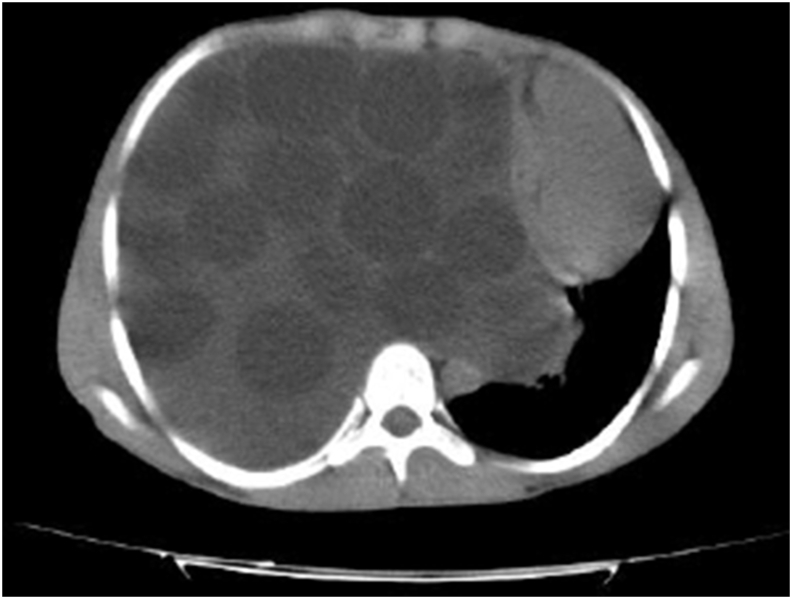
Patient's CT scan: multiple round-shaped cystic formations filling up the entire right pleural cavity.
